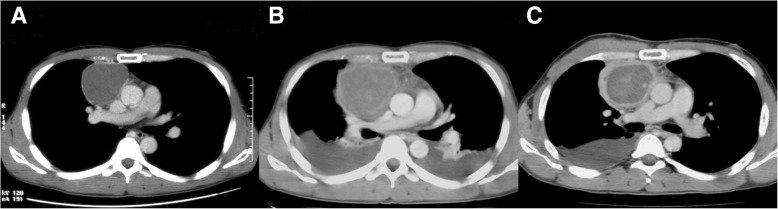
a Enhanced chest computed tomography scan before hospitalization showing the giant cystic mediastinal tumor. The inside of the cyst is homogeneous, and the surroundings are enhanced. b Enhanced chest computed tomography scan on hospitalization day 2 showing the expansion and heterogenization of the cystic tumor with bilateral pleural effusion. c Enhanced chest computed tomography scan on hospitalization day 14 showing the reduction of pleural effusions, thickening of the cystic capsule, reduction of the tumor’s size, and re-homogenization of the cystic tumor
